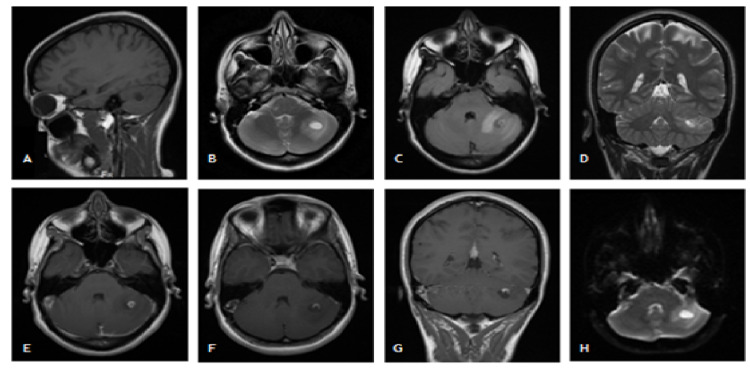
Encephalic MRI showing imaging compatible with left cerebellar hemisphere hemangioblastoma. Rounded lesion, hypointense on T1 and hyperintense on T2, with thick signal reinforcement after gadolinium, approximately 7 mm in diameter, located in the left cerebellar hemisphere; inferiorly to the “ring” lesion, a cyst measuring approximately 12.5 mm in diameter, whose interior weights homogeneously like cerebospinal fluid (CSF); larger area of ​​surrounding edema compared to previous examinations. T1-weighted sagittal plane (A), T2-weighted axial plane (B), T2-weighted axial fluid-attenuated inversion recovery (FLAIR) plane (C), T2-weighted coronal plane (D), T1-weighted axial plane after intravenous administration of gadolinium contrast (E) and (F), T1-weighted coronal plane after intravenous administration of gadolinium (G), axial plane diffusion imaging (H). MRI: magnetic resonance imaging.
